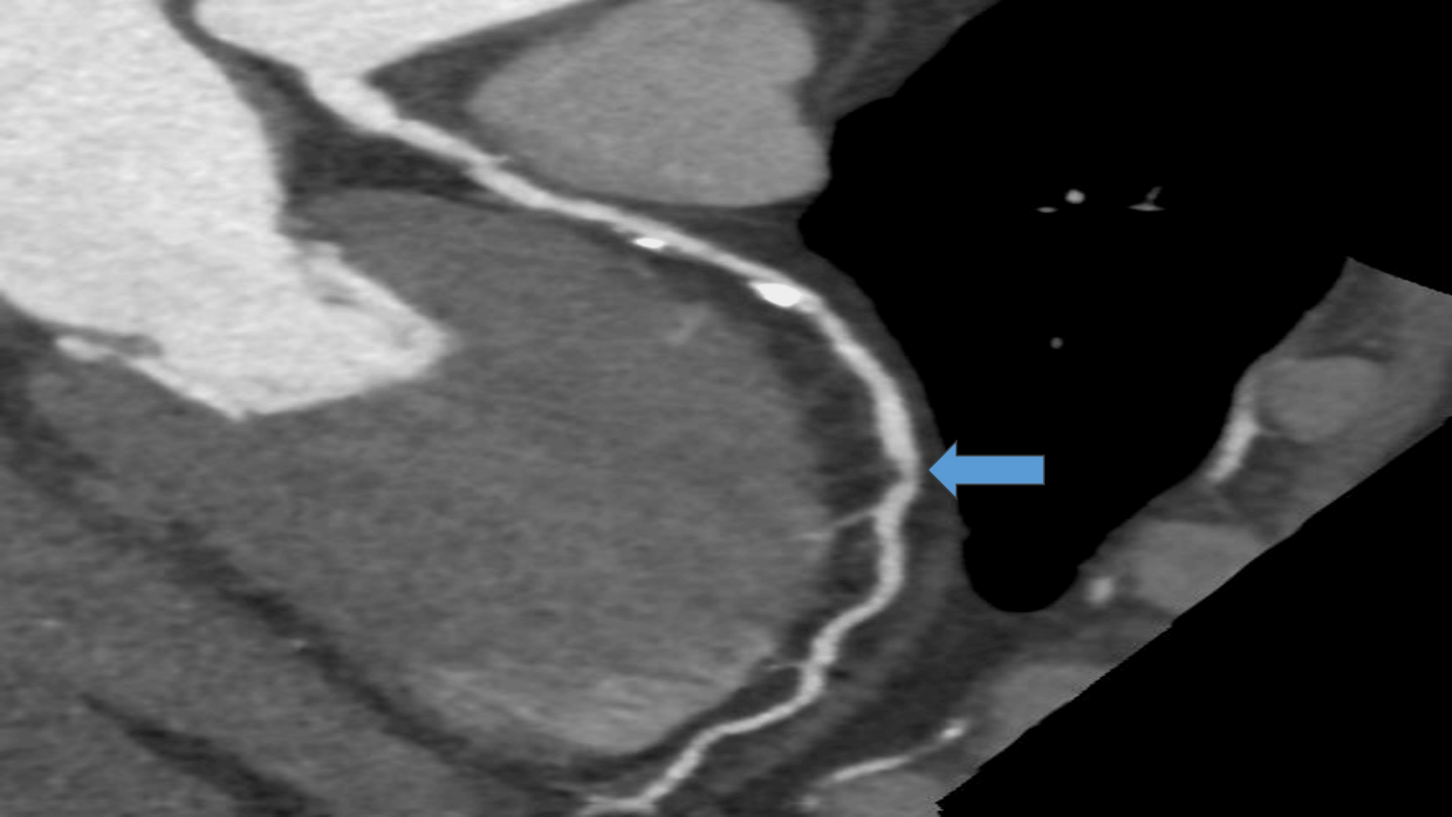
CT-Coronary Angiogram The arrow indicates moderate stenosis in the left anterior descending artery CT, Computed Tomography
